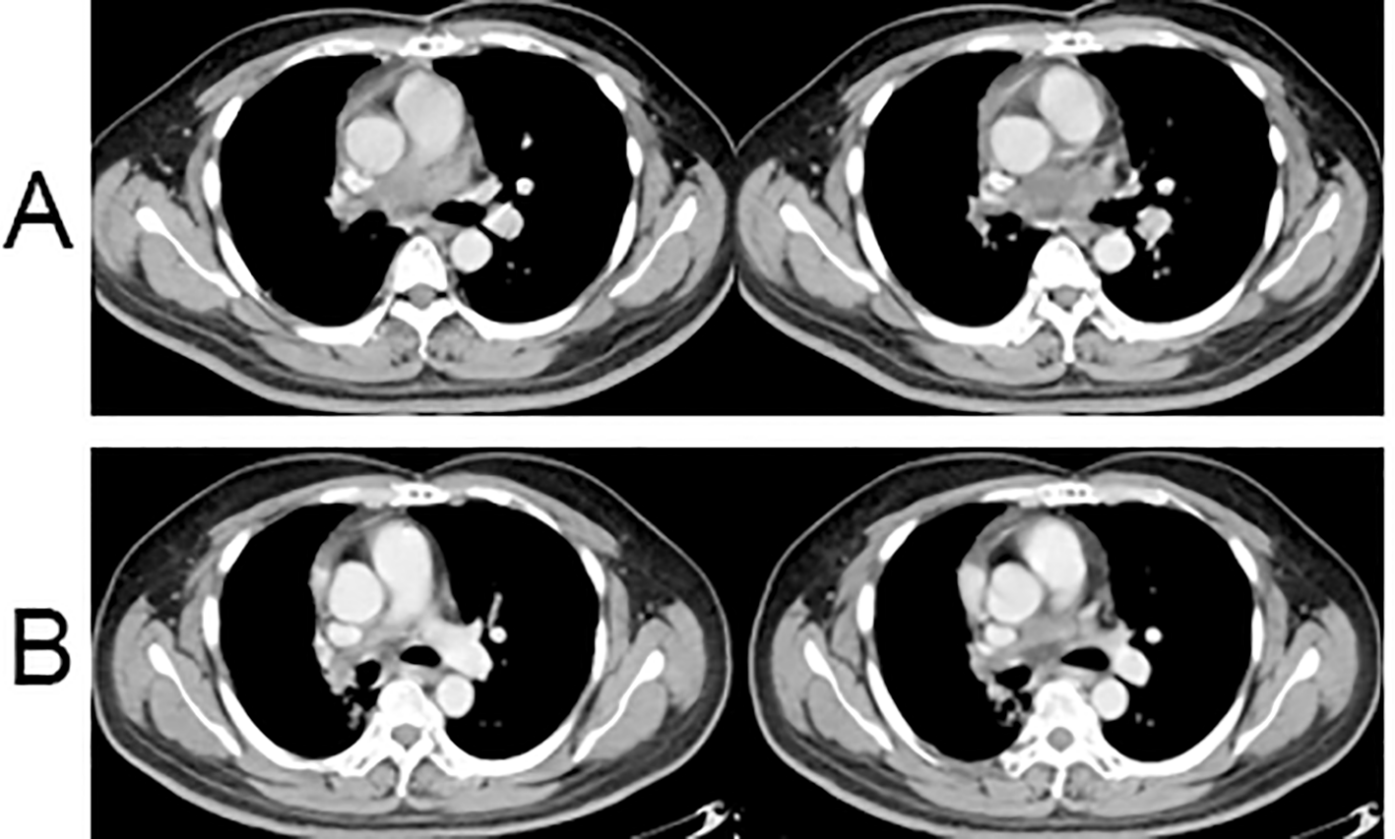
Postoperative chest CT results. (A) Enhanced chest CT scan at 1 month after surgery; (B) Enhanced chest CT scan at 6 months after surgery.
